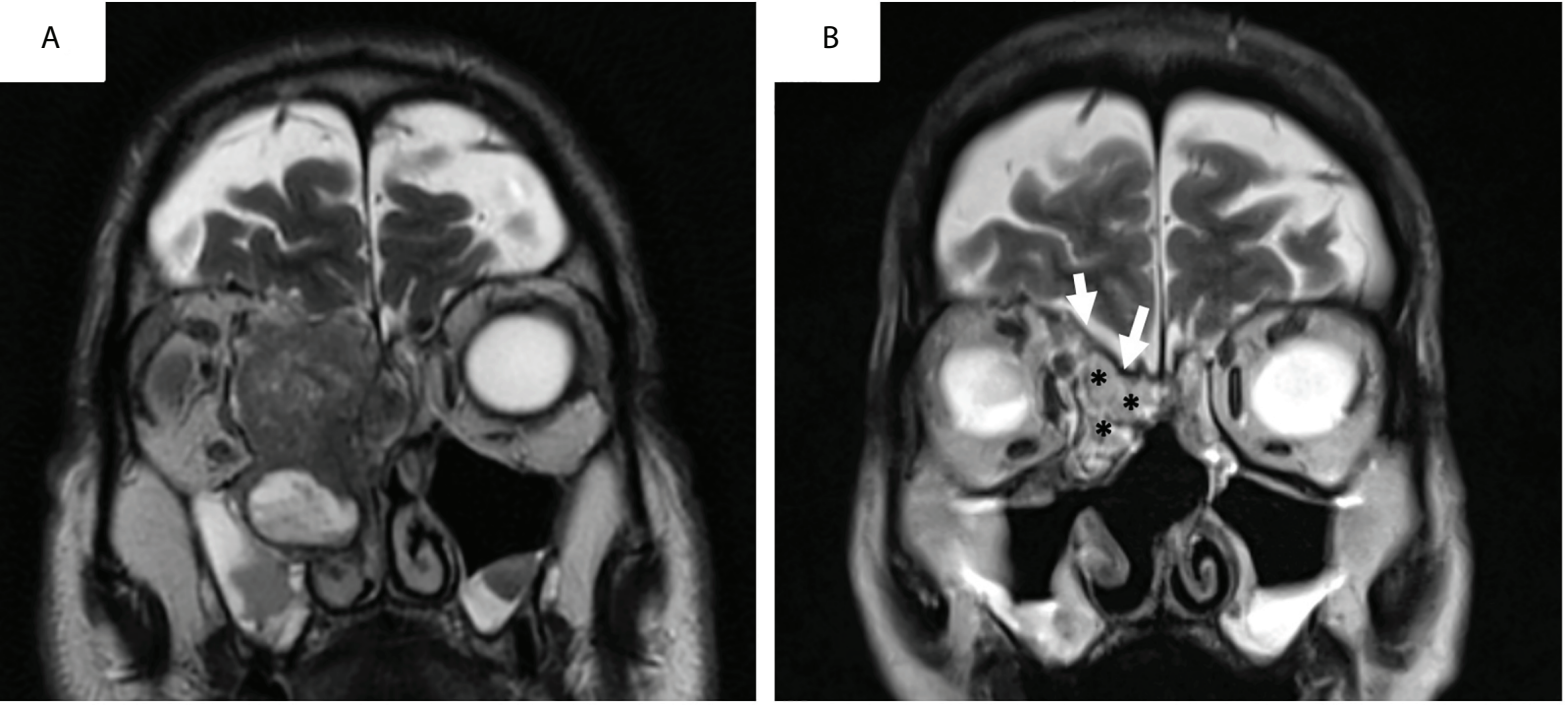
Pre-operative (A) and early post-operative aspect (postoperative day5) of a skull base reconstruction with fascia-lata and abdominal fat graft (B) for an intestinal type adenocarcinoma on T2-weighted MRI sequences.
